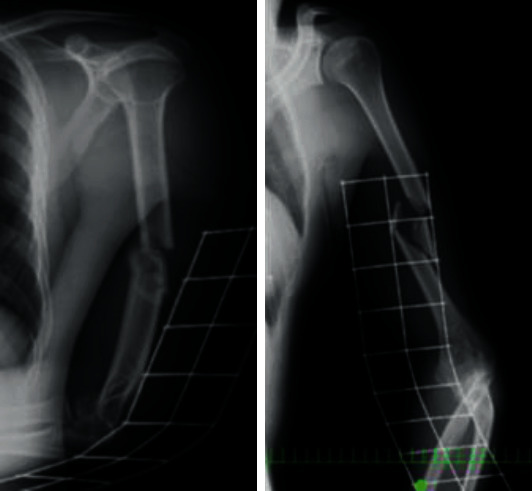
Radiographs of a left humeral shaft fracture. A fracture can be seen with the third and fourth fragments in the mid shaft. The AO-OTA classification of this fracture is 12-C3.
